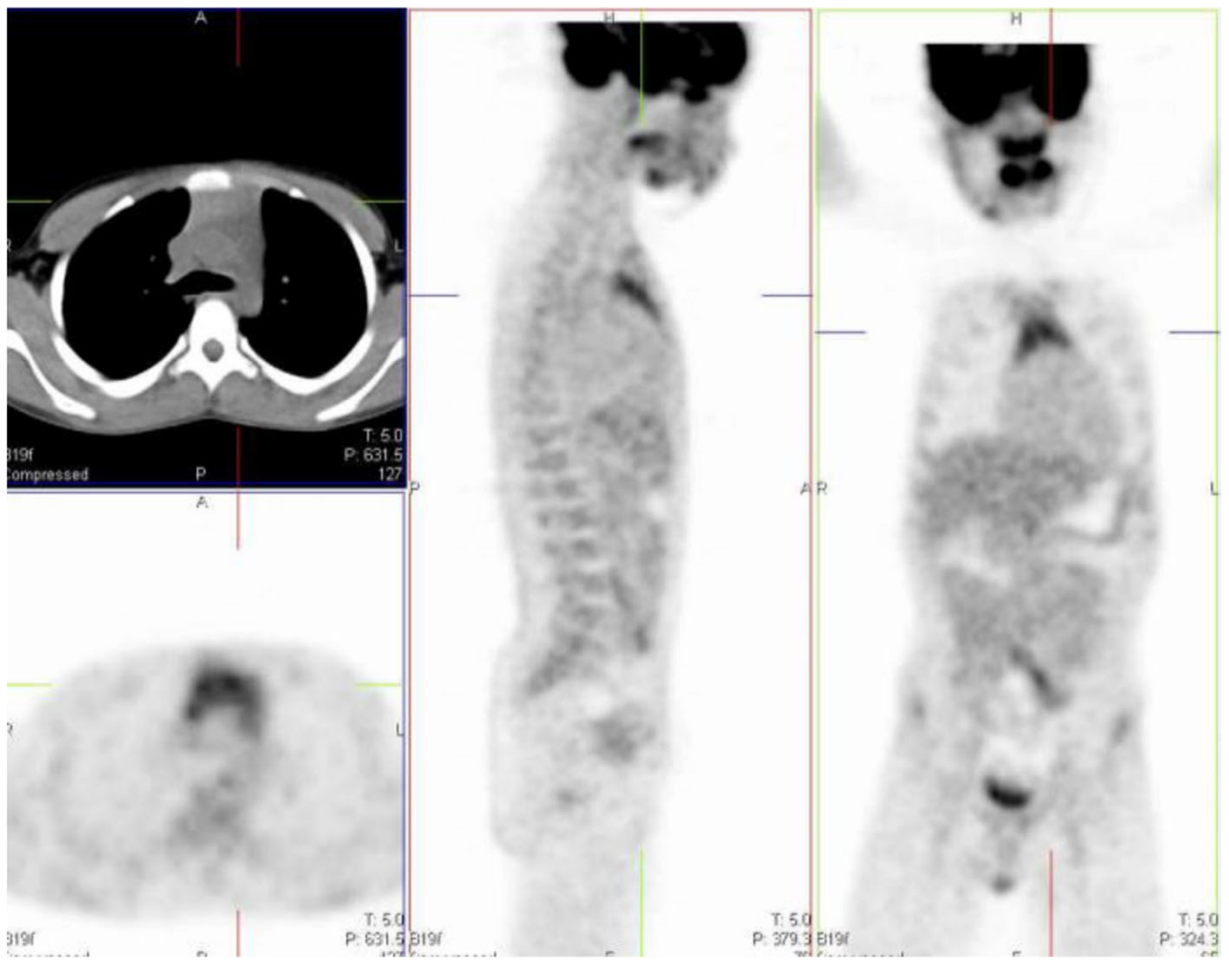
12 year old male referred for the evaluation of active vasculitis. FDG PET study showing diffuse symmetrical increased uptake in the thymus seen on the axial, sagittal and coronal views (inverted ‘'V”).
